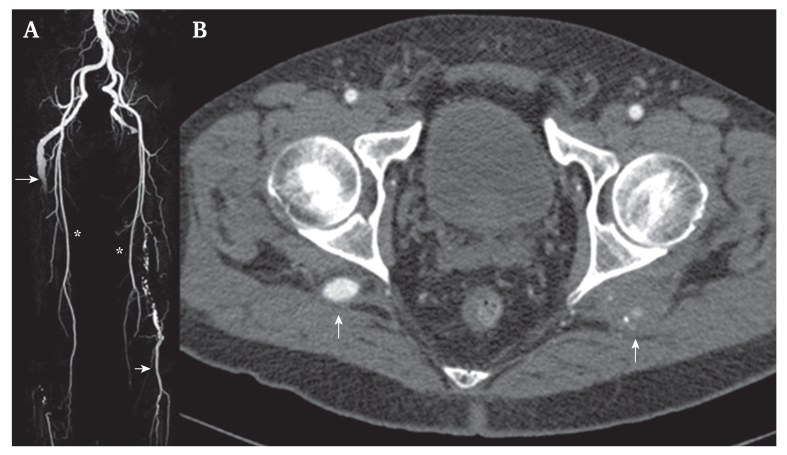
(A) Coronal maximum intensity projection and (B) axial computed tomography images showed persistent bilateral sciatic artery (arrows) affected by occluded aneurysmal dilatations, small calibre bilateral superficial femoral arteries (stars), and patent left side popliteal and tibial vessels (arrow).
